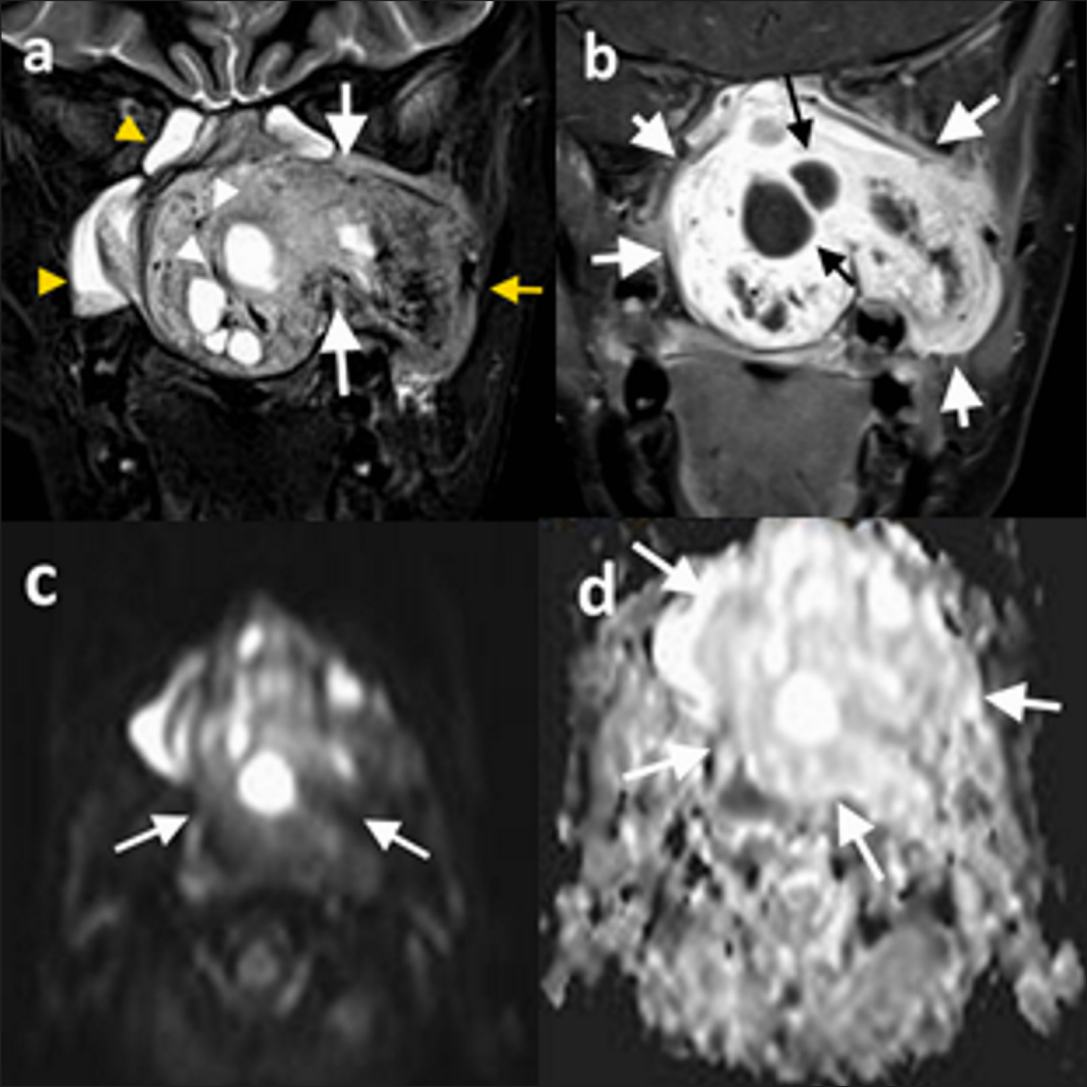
13-year-old male patient (patient 5), (a) coronal T2-weighted precontrast, (b) coronal T1-weighted postcontrast, (c) DWI, and (d) ADC map demonstrate a left-sided nasopharyngeal mass which enlarges the ipsilateral pterygopalatine fossa (*a, white arrows*) and extends into the temporal fossa (*a, yellow arrow*). The mass is hyperintense on T2-weighted image (a) and exhibits significant contrast enhancement (b). Diffusion-weighted images (c) showed no diffusion restriction, and the lesion has high signal intensity on the ADC map (d). The tumor demonstrates internal cystic components (*b, black arrows*) and signal-void regions (*a, white arrowheads*). Image a demonstrates inflammatory signal changes in maxillary and sphenoid sinuses (*yellow arrowheads*).
